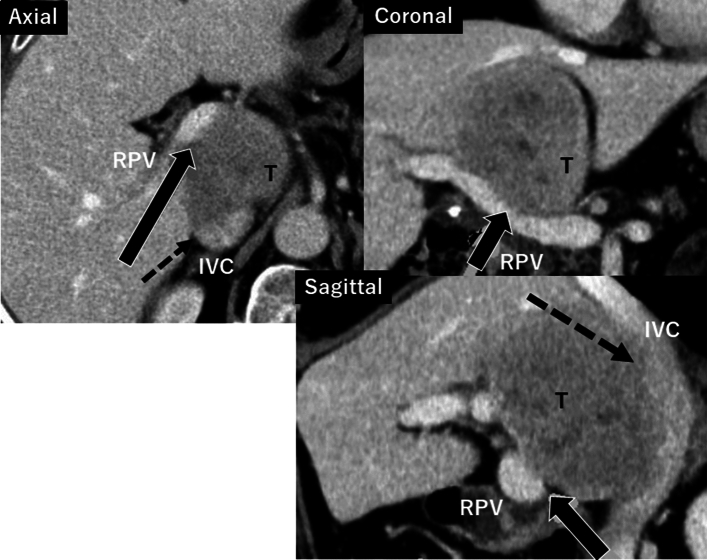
The solitary 6 cm-in-size of HCC was observed in the segment 1 by the enhanced CT findings. At the venous phase, the entire tumor enhancement was washed-out and compressed the portal trunk (black arrow), posterior portal pedicle and inferior vena cava (black dotted arrow), which was supposed to be vascular involvement
